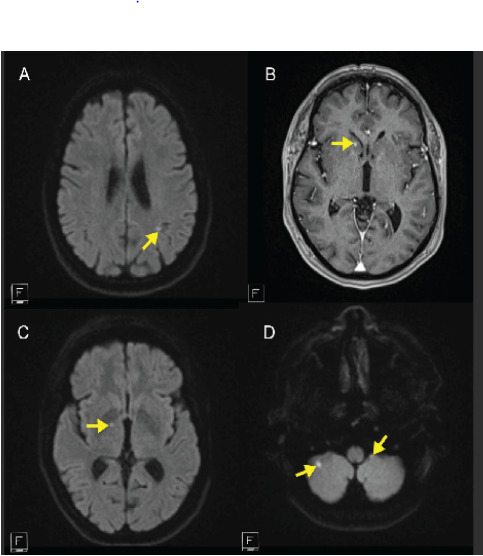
Axial images of brain magnetic resonance imaging obtained with T2-weighted flair (A), T1-weighted post contrast (B), and diffusion-weighted (C, D) sequences showing punctate hyperintensities in the periventricular and deep white matter and cerebellum (yellow arrows) consistent with chronic, small-vessel ischemic changes.
